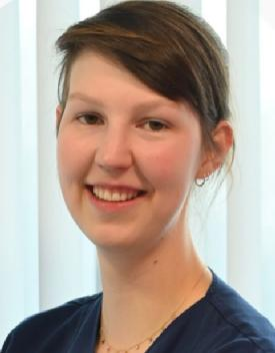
Dr Margaretha Van Kerrebroeck earned her medical degree with great distinction from the Catholic University of Leuven in 2018. She is currently a trainee in cardiology at the University Hospitals of Leuven with a specific focus on heart failure, echocardiography, and intensive care.
